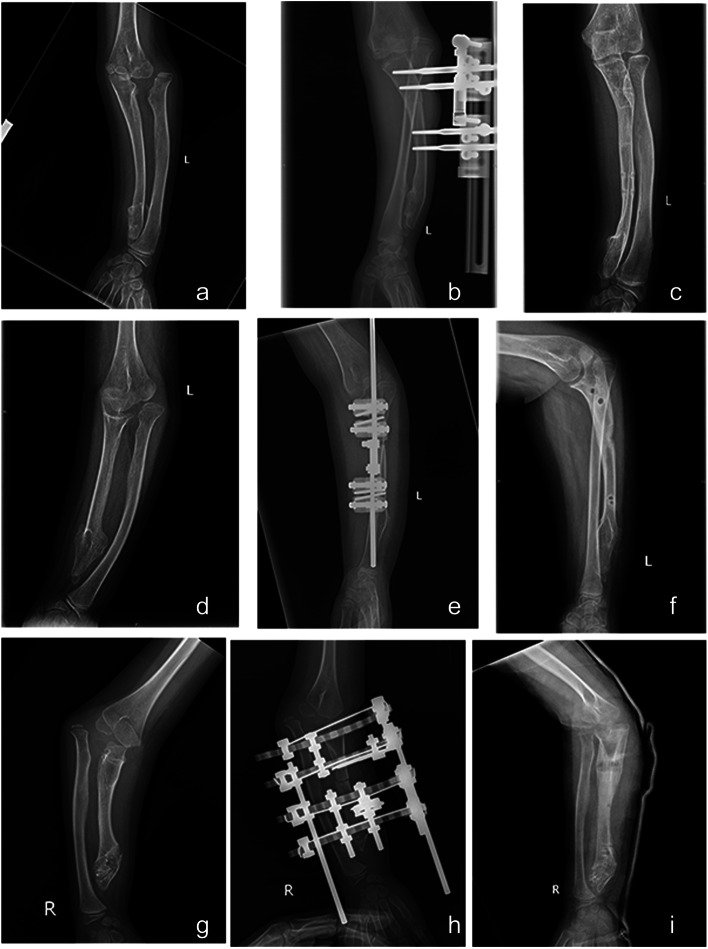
a-c Pre-operative and post-operative radiographs of Patient 1. d-f Pre-operative and post-operative radiographs of Patient 8. g-i Pre-operative and post-operative radiographs of Patient 4
